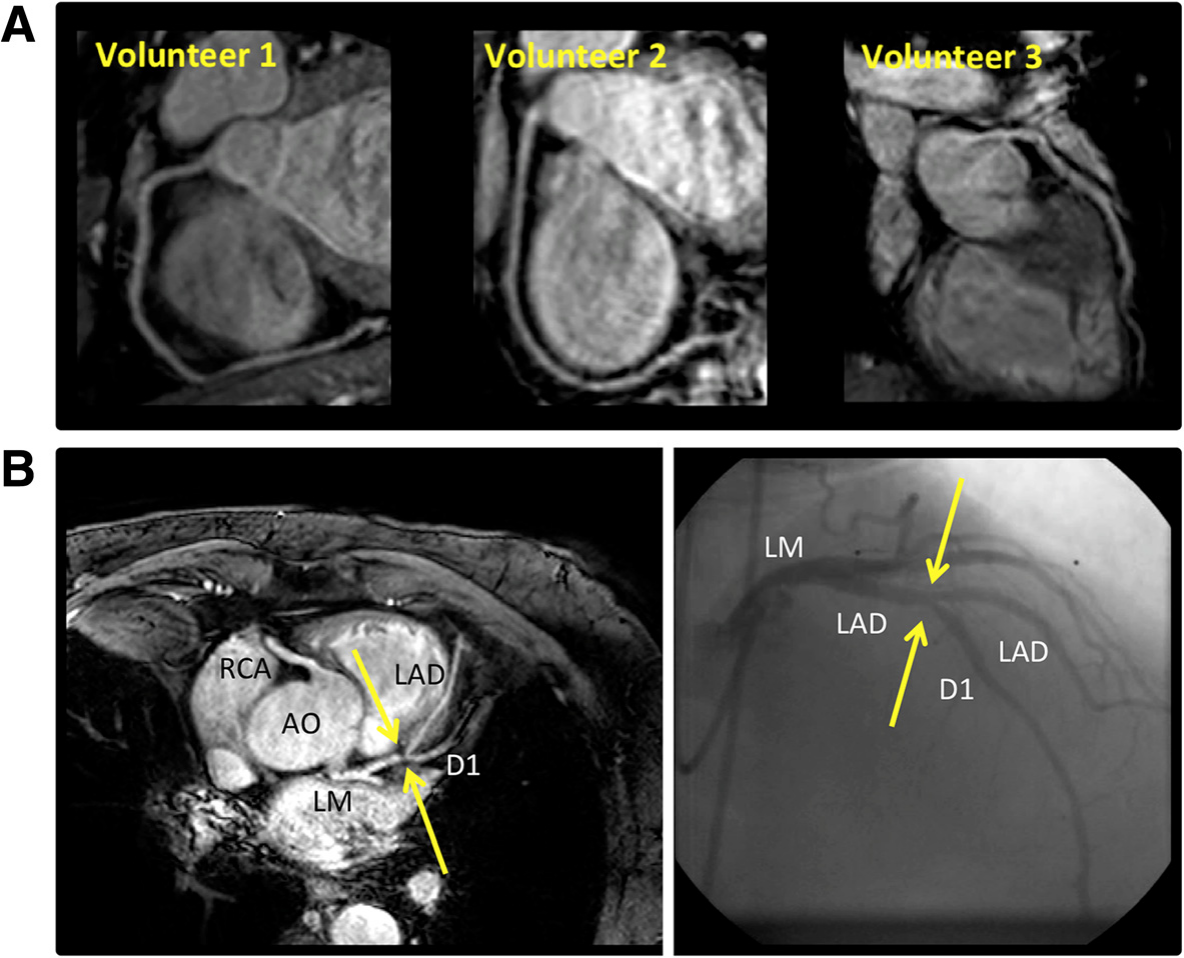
Double oblique single-breathhold 3D bSSFP coronary magnetic resonance angiograms of the right coronary artery and left anterior descending in three healthy volunteers (A) and in a 66-year-old patient (B) with 50-70% lesion at the diagonal branch of the LAD identified using diagnostic x-ray angiogram. AO: Aorta, RCA: Right Coronary Artery. LM: Left Main Artery. LAD: Left Anterior Descending Artery. D1: First Diagonal Branch. MRA Images were multi-planar reformatted.
